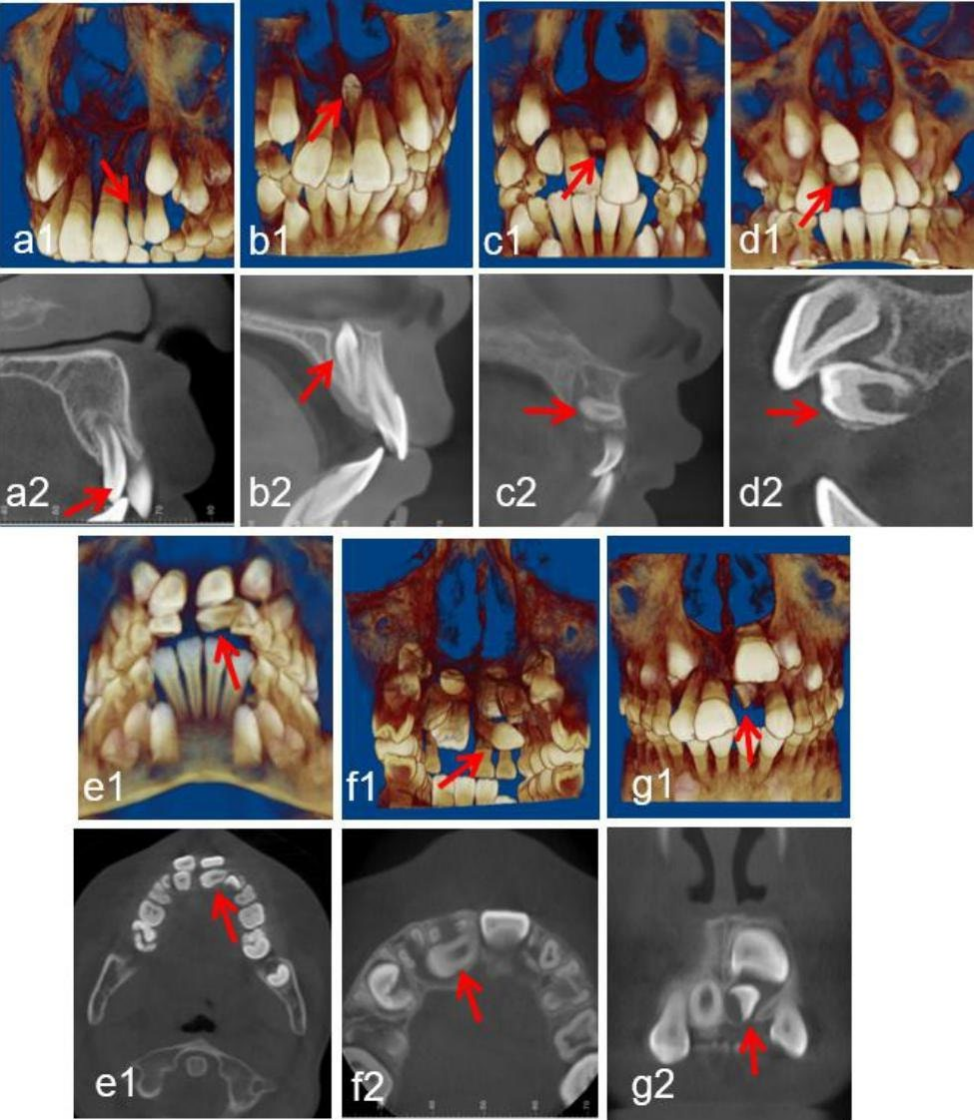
Representative CBCT images exhibiting diverse orientation of ST. (a1-a2) normal; (b1-b2) inverted; (c1-c2) palatal transverse; (d1-d2) labial transverse; (e1-e2) mesial horizontal; (f1-f2) distal horizontal; (g1-g2) undefined. The red arrows point to the ST in the CBCT images
